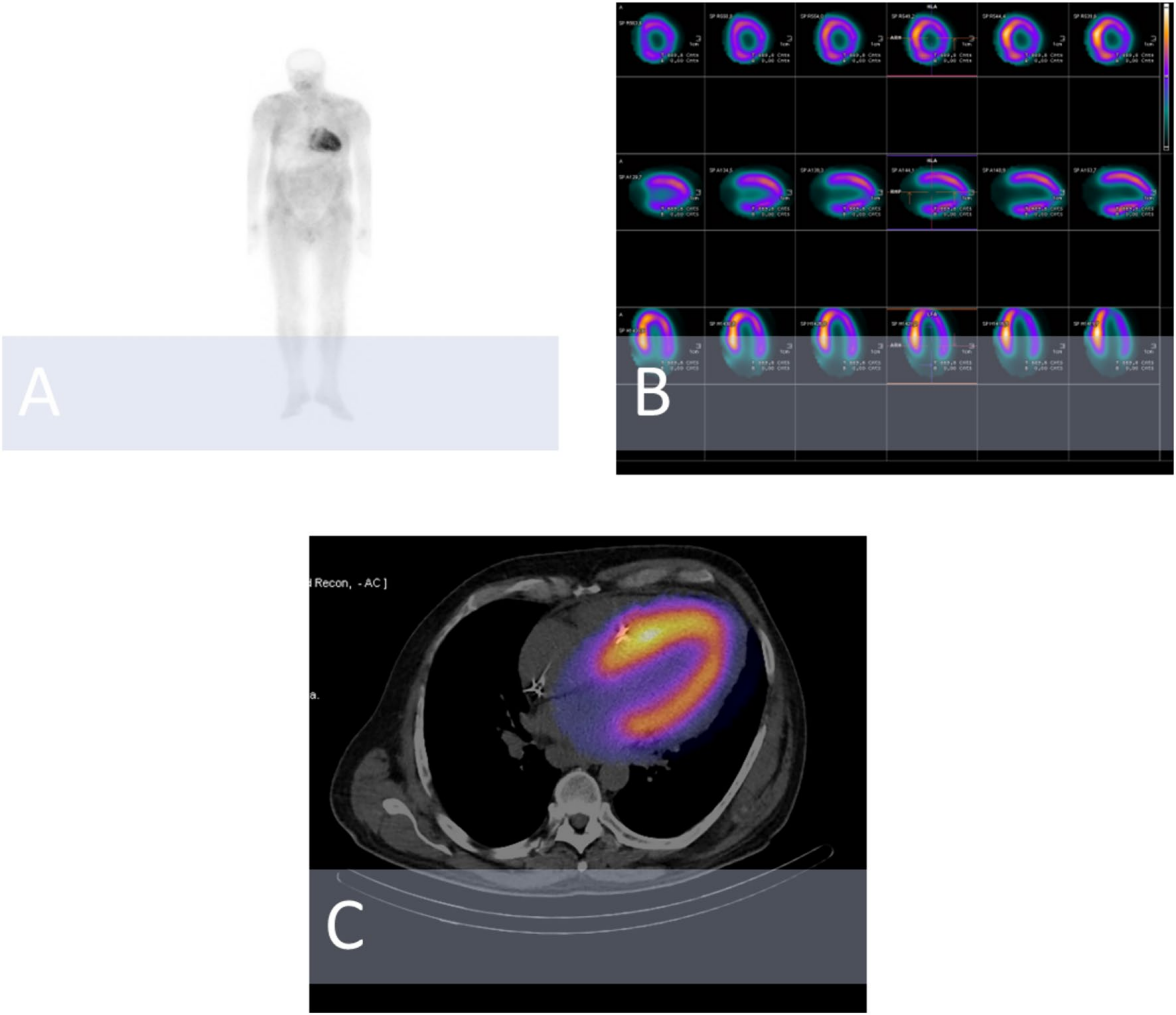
Findings in the course of hereditary cardiac transthyretin amyloidosis. A planar whole-body scintigraphy with [99mTc]Tc-DPD (grade 3). B SPECT imaging with [99mTc]Tc-DPD (after attenuation correction). C hybrid SPECT/CT imaging. *CT* computed tomography, *DPD* 3,3-disphono-1,2-propanodicarboxylic acid, *SPECT* single-photon emission computed tomography
